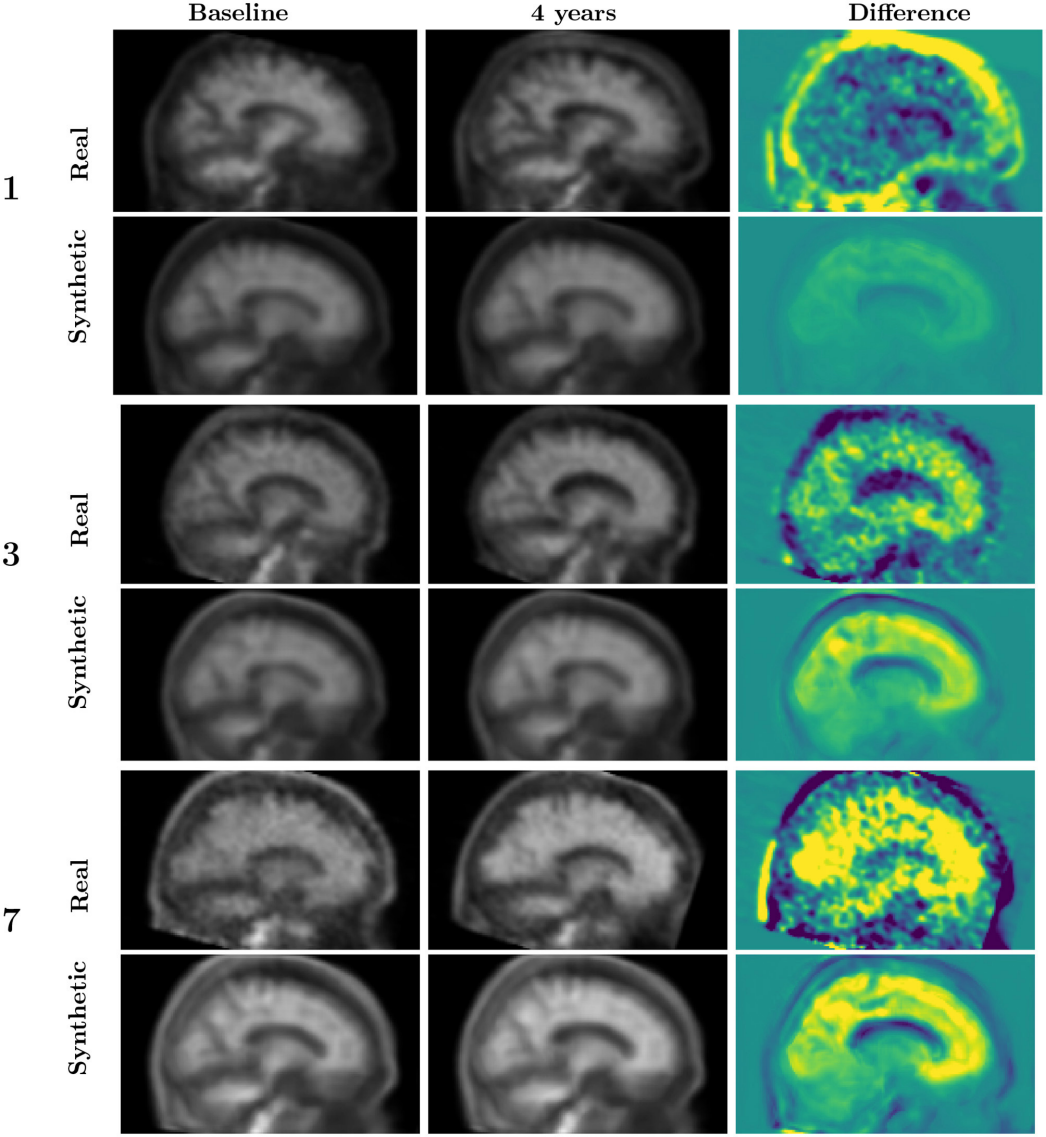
Synthetic vs. observed 4-year progression for participants experiencing brain amyloid accumulation. Participant 1 has a lower rate of SUVR increase than participand 3 and 7. The image range is from 0 to 4 for the gray images (first and second rows) and from −0.4 to 0.4 for the colored images (third rows), where blue corresponds to more negative values, yellow to positive values and green to zero. See Figure 4 for the corresponding latent representation.
